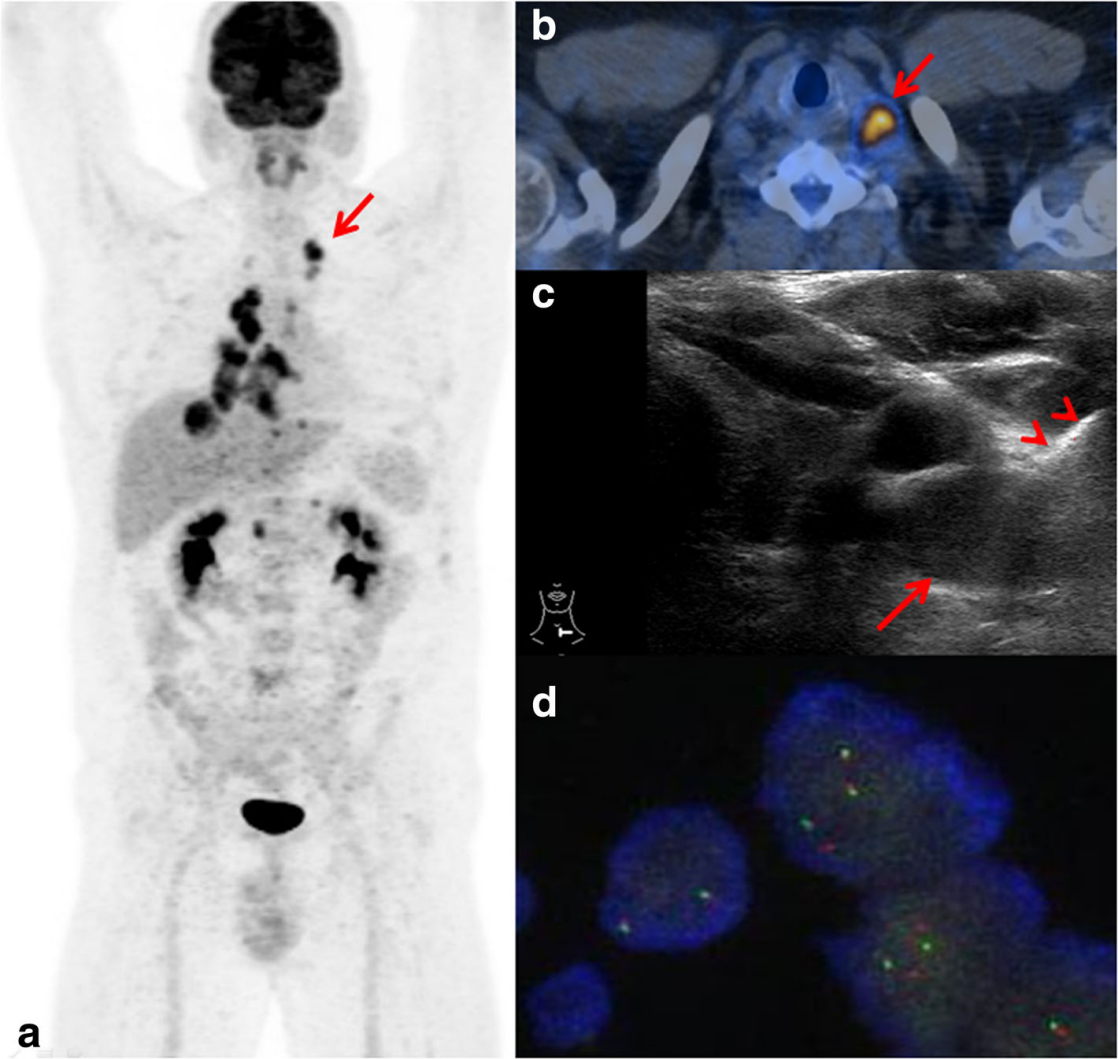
FDG PET/CT of a patient with suspected metastatic lung cancer. Maximum intensitiy projection image a showed primary lung cancer in the right lower lobe with extensive hilar, mediastinal, bilateral supraclavicular (*arrow*) and abdominal lymph node metastases. Small bone metastases e.g. in the left iliac bone with low metabolic activity were visible. In MR (not shown) multiple brain metastases were detected. Fused axial PET/CT image b showed FDG active left sided SCLN (*arrow*). Ultrasound guided FNAC and CB documentation (*arrowheads*) (c). Molecular testing showed ALK rearrangement d and the patient was treated with brain radiation and crizotinib
